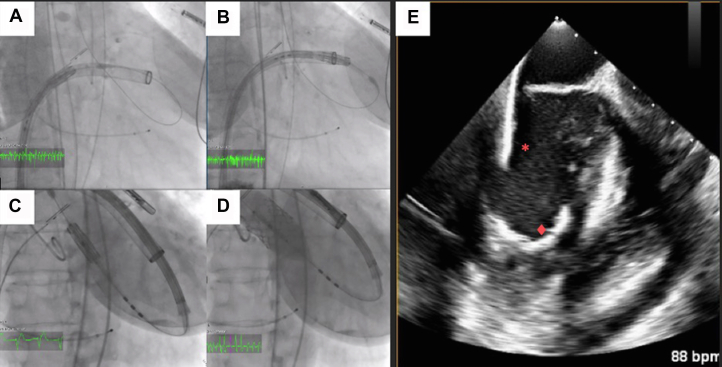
Prosthetic Aortic Valve Delivery Fluoroscopic images of the Edwards Commander delivery system loaded with the prosthetic valve within the DRYSEAL sheath (A), at the distal end of the sheath (B), within the aortic valve apparatus (C), and at deployment (D). Intracardiac echocardiographic images show the DRYSEAL sheath in the left ventricular midcavity (∗) with the prosthetic valve (♦) at the apex curving superiorly toward the aortic outflow tract (E).
